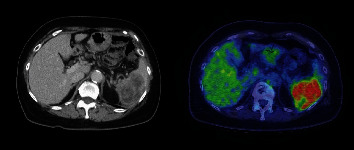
CT and PET-CT of recurrent ovarian cancer. A solid mass occupies the spleen.
